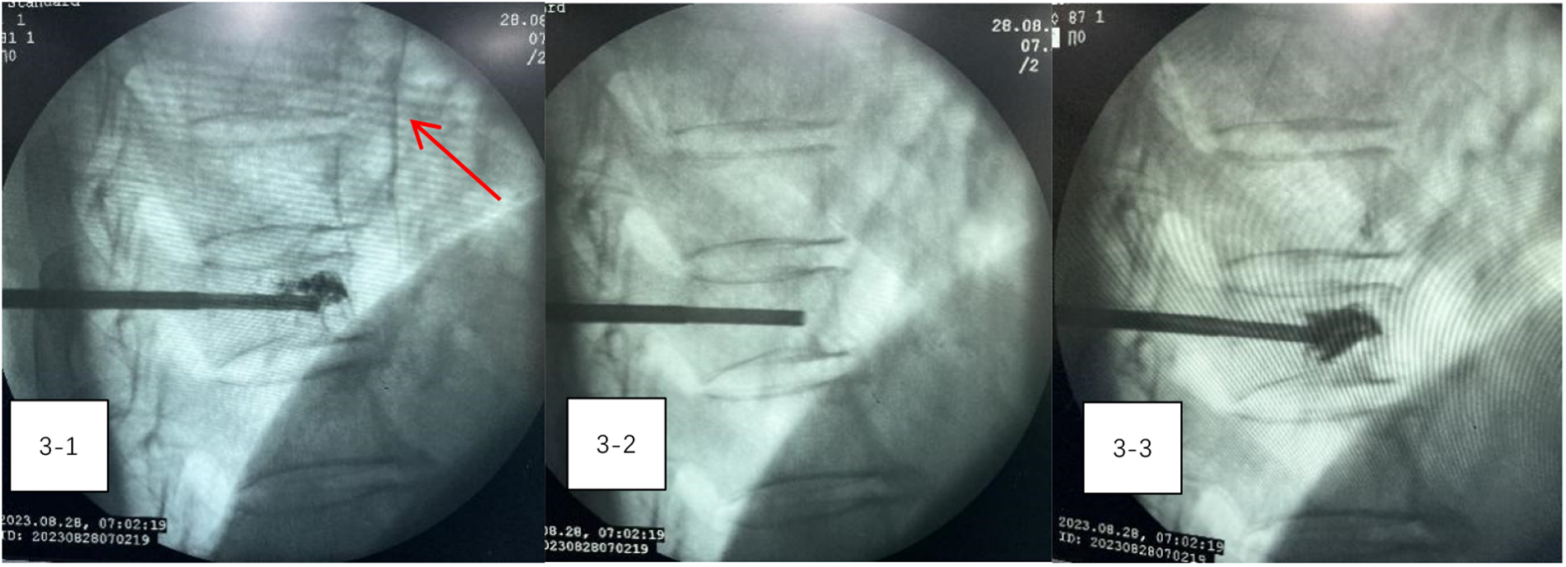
(3-1–3-3) A comparison of pre-and post-injection x-rays showed no elongated high-density shadow before the injection, which appeared after re-injecting 0.5 ml of cement.
